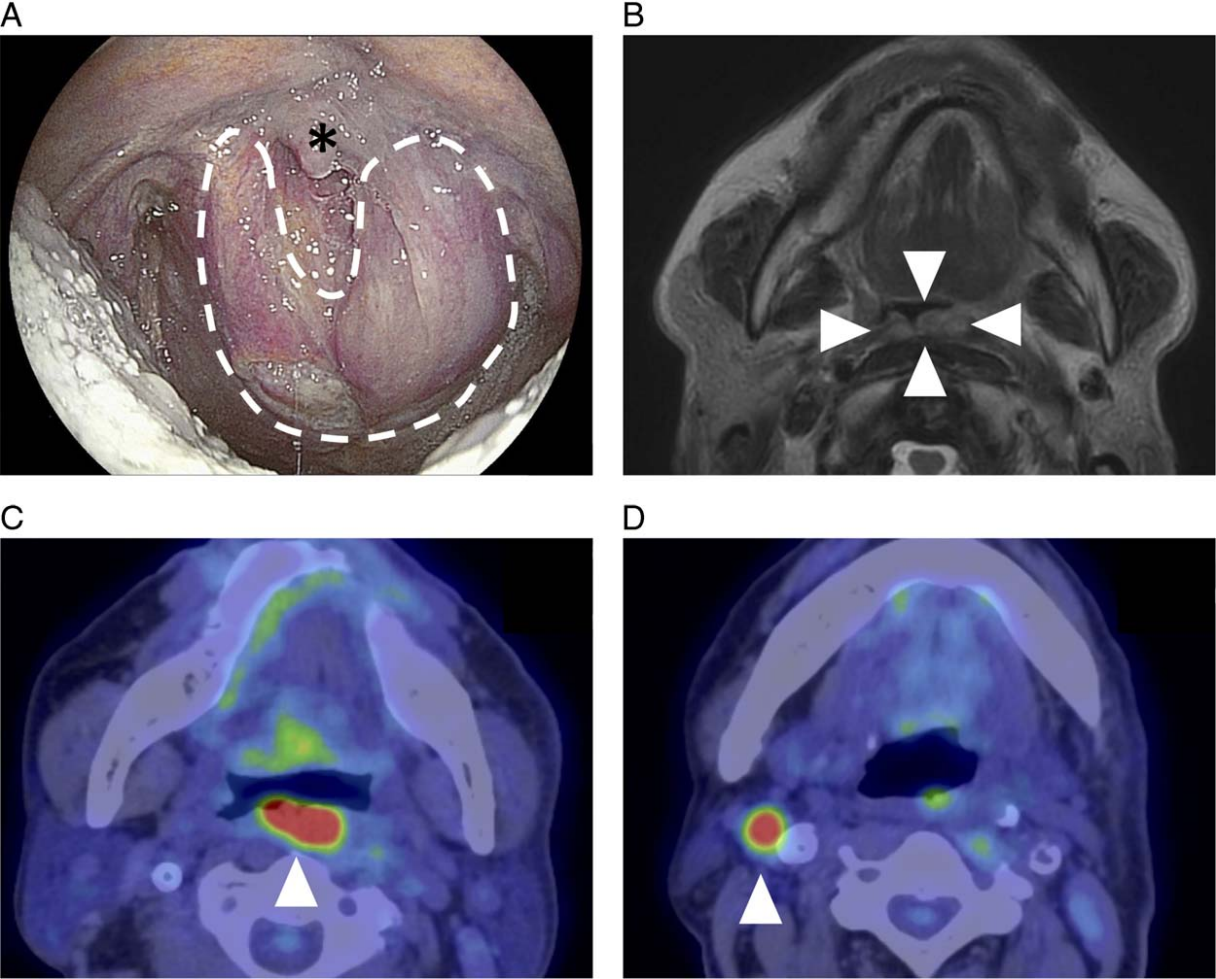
Preoperative images. (A) Endoscopic examination. The precise extent and outline of the tumor are obscure. A heart-shaped tumor (dotted line) is seen. *, uvula. (B) T2-weighted MRI imaging, a recurrent tumor (arrowheads) is seen in the oropharynx. (C, D) FDG-PET/computed tomography imaging. Recurrent lesions (arrowheads) are seen in the oropharynx (C) and right cervical lymph node (D).
